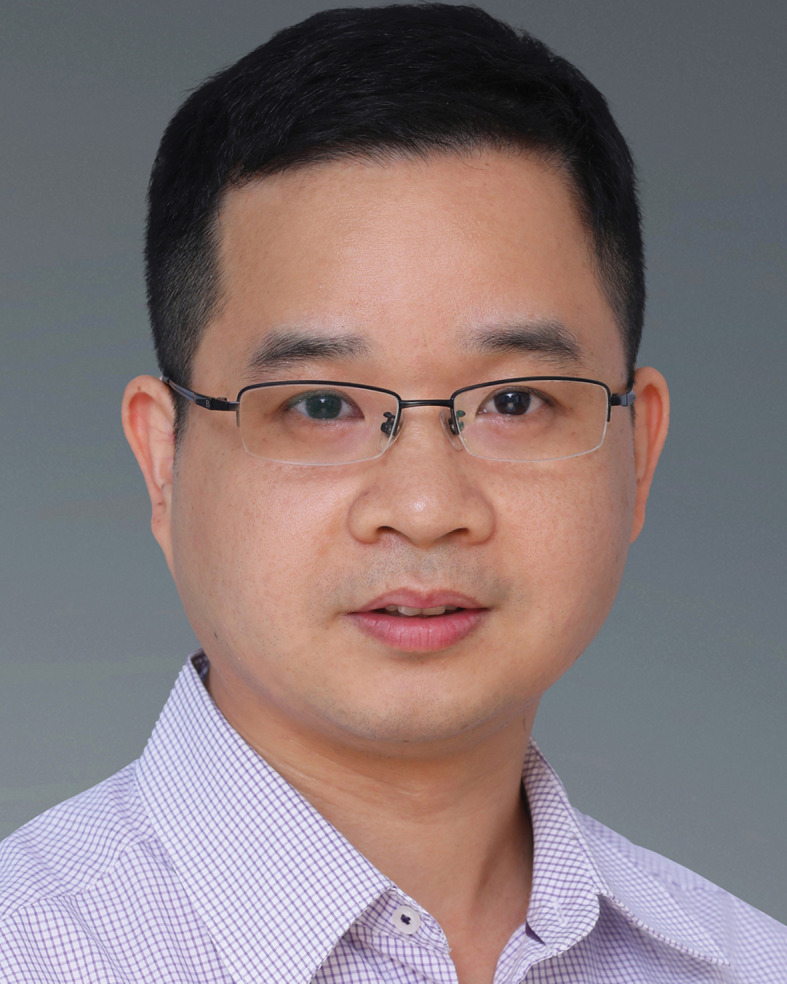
Jinhao Gao obtained his BSc from Nanjing University in 2004 and his PhD from The Hong Kong University of Science and Technology (HKUST) in 2008. He was a postdoctoral fellow at Stanford University from 2008 to 2010. He is now a professor of chemical biology at Xiamen University. He was awarded the National Natural Science Fund for Excellent Young Scholars (2012) and Distinguished Young Scholars (2021). His main research interests include chemical biology, nanochemistry, MRI probes, molecular imaging, and cancer therapy.
